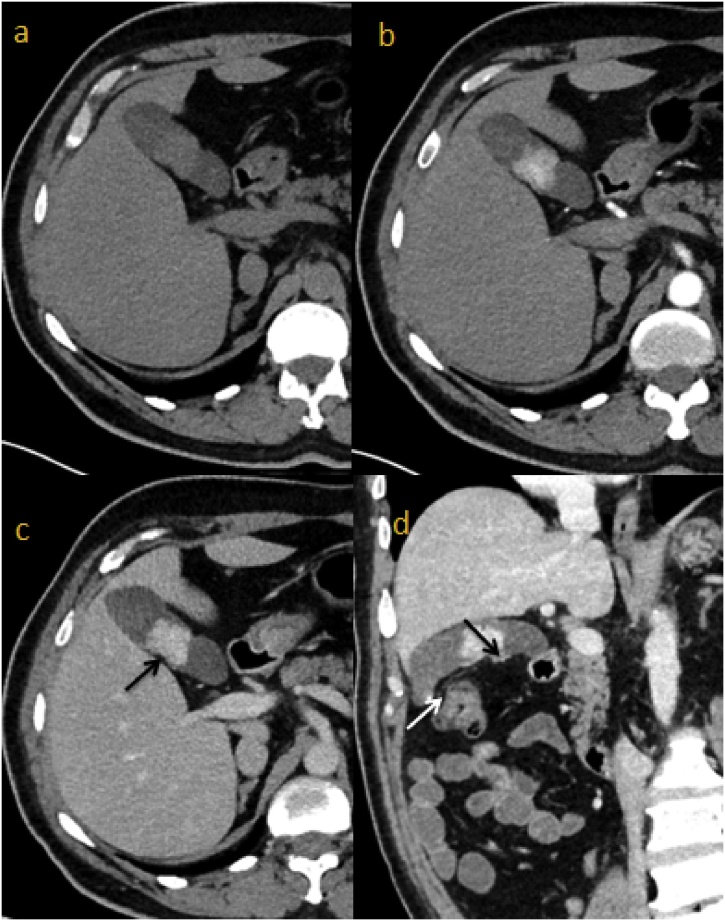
Abdominal thin slices CT scan in axial plan : non enhanced (a), arterial (b) and portal acquisition (c). Coronal oblique thin slice CT scan (d): Lobulated density mass, in the gallbladder, with intense arterial enhancement after contrast injection, and retracting gallbladder wall (black arrows). It was associated with gallstones (white arrow).
